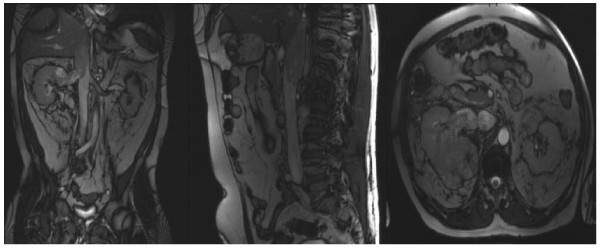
MRI images of a level IV disease.
